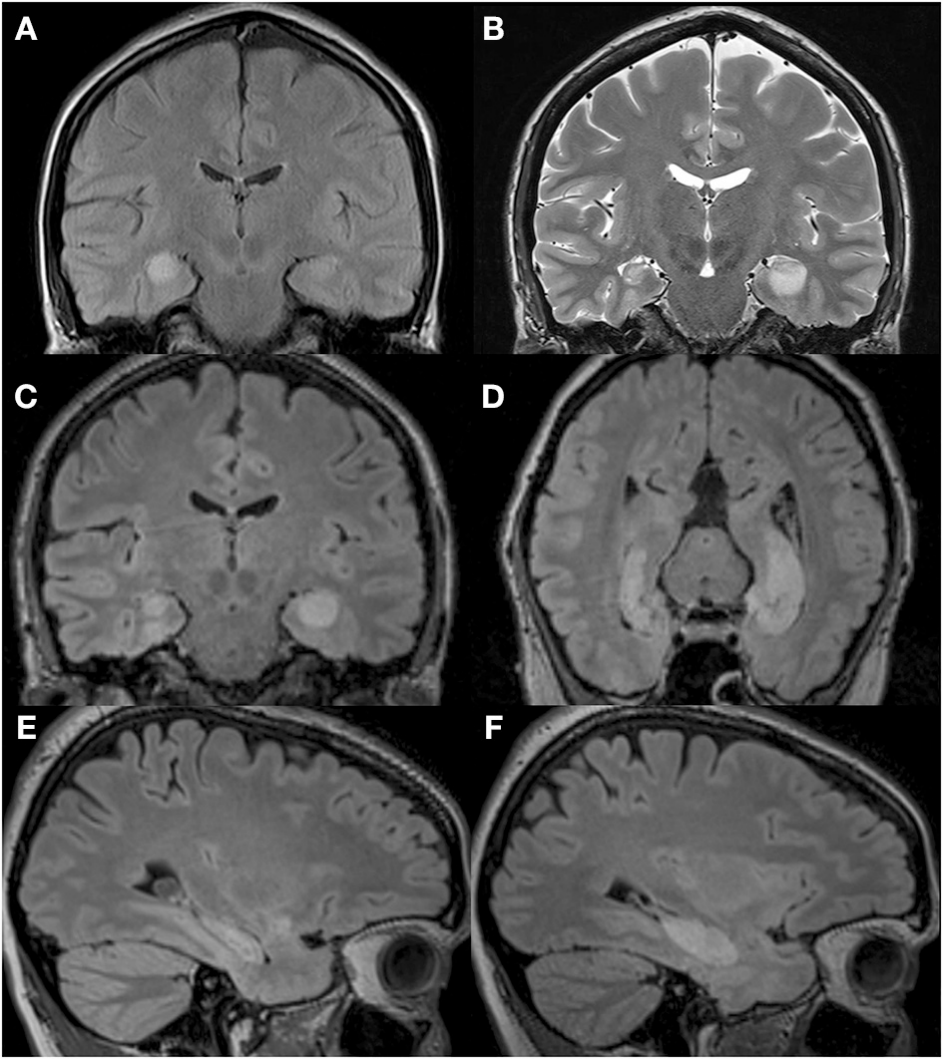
T2-weighted MRI images of P9's brain showing the extent of the hypersignal reflecting bilateral hippocampal abnormality. (A) MRI performed upon admittance to the hospital following a generalized tonic-clonic epileptic seizure revealed signs of edema over the right hippocampus. (B) MRI performed 2 months following the initial epileptic episode revealed signs of edema over the left hippocampus, and a shrunken right hippocampus. Reproduced from Petel et al. (2011), with permission. (C–F) T2-weighted MRI images of P9's brain performed 2.5 months after the initial episode and showing a shrunken right hippocampus in three different planes: coronal (C), parallel to the long axis of the hippocampus (D) and sagittal (E), and a hypersignal restricted to the left hippocampus in three different planes: coronal (C), parallel to the long axis of the hippocampus (D) and sagittal (F).
